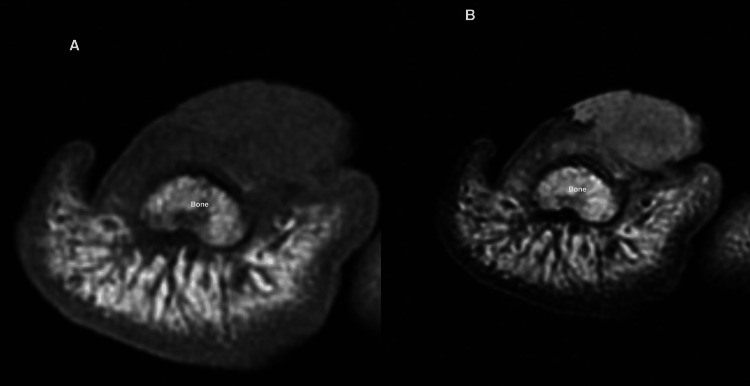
MRI of squamous cell carcinoma of the nail unit A: axial MRI of the nail in a patient diagnosed with squamous cell carcinoma, with the goal to assess the bone extension and the T1-weighted image shows an exophytic mass that is moderately hypointense, involving the entire nail bed and lateral nail fields; B: T1-weighted image with contrast enhancement, which allows for precise delineation of the hyperintense mass. The contrast enhancement confirms that there is no intraosseous extension. Original unpublished image, courtesy of Dr. Claudia Gonzalez.
